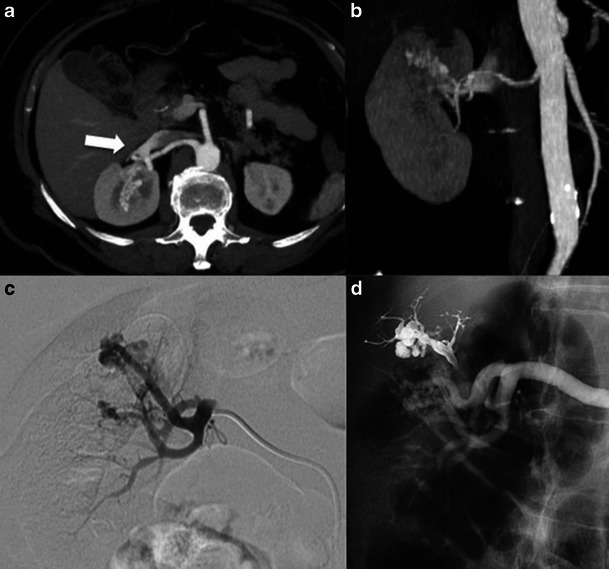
A 72-year-old woman was admitted to the emergency department because of right flank pain and macroscopic haematuria. Laboratory investigation revealed haemoglobin of 9.3 mg/dl. Abdominal US showed only multiple clots within the urinary bladder. a-b Contrast-enhanced CT scan revealed a conglomerate of vessels in the upper pole of the right kidney, visible in arterial phase with early filling of the IVC (arrow). The diagnosis of RAVM was made and the patient was submitted to a selective right renal angiogram using a hydrophilic 4-F Simmons 1 catheter. c Angiogram showed a tortuous nidus in the RAVM at the level of the upper renal pole with supply from two different superior branches without contrast blush, which would have suggested active bleeding. d Subsequent embolisation with injection of liquid embolic material (Onyx, eV3 Europe SAS, Paris, France); interval angiogram shows exclusion of the upper lesion. Angiogram of the lower lesion followed
